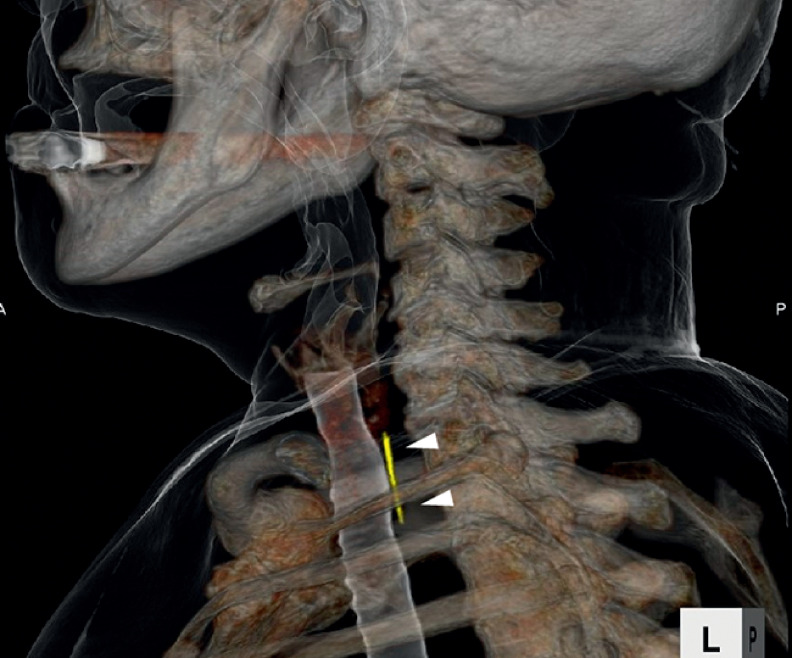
Computed tomography image showing a 25-mm fishbone (arrowheads) in the upper esophagus.
